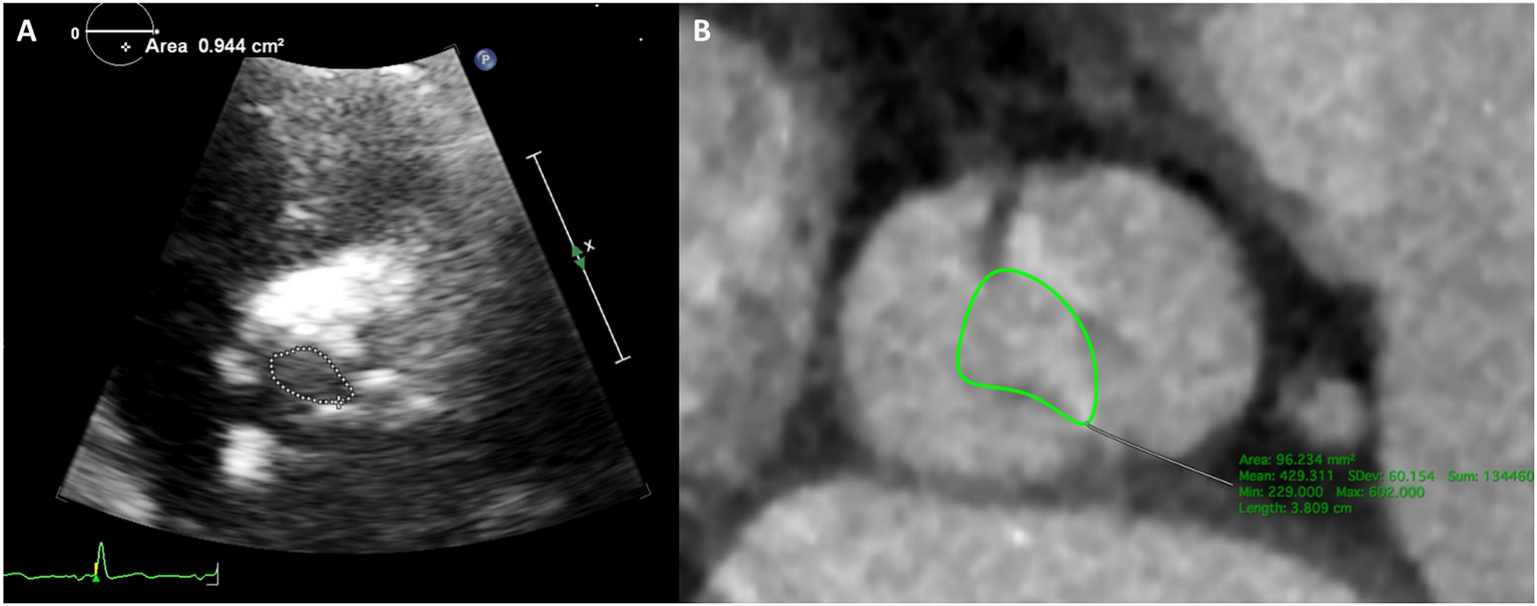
Type-0 bicuspid aortic valve orifice area measured by echocardiography and CT. (A) Aortic valve orifice area was 0.94 cm^2^ measured by TTE. (B) Aortic valve orifice area was 0.96 cm^2^ measured by CT of systolic phase.
